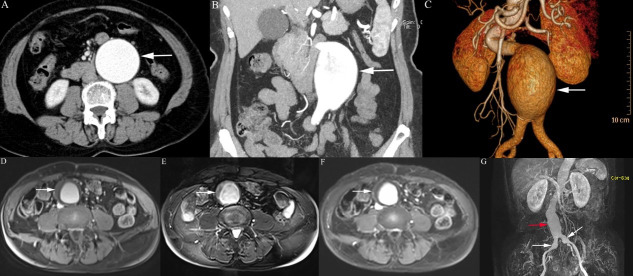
Multimodality imaging assessment of abdominal aortic aneurysms. (A) Axial CTA image, (B) coronal maximum intensity projection (MIP), and (C) volume-rendered (VR) reconstruction demonstrating an infrarenal AAA (white arrows) with clear visualization of the aneurysm neck and iliac arteries. (D) Axial T1-weighted image (T1WI), (E) T2-weighted image (T2WI), and (F) contrast-enhanced T1WI showing an infrarenal AAA (white arrows) with a filling defect consistent with intraluminal thrombus. (G) Coronal three-dimensional reconstruction image reveals the AAA (red arrow) and bilateral iliac artery aneurysms (white arrows). CTA, computed tomography angiography.
